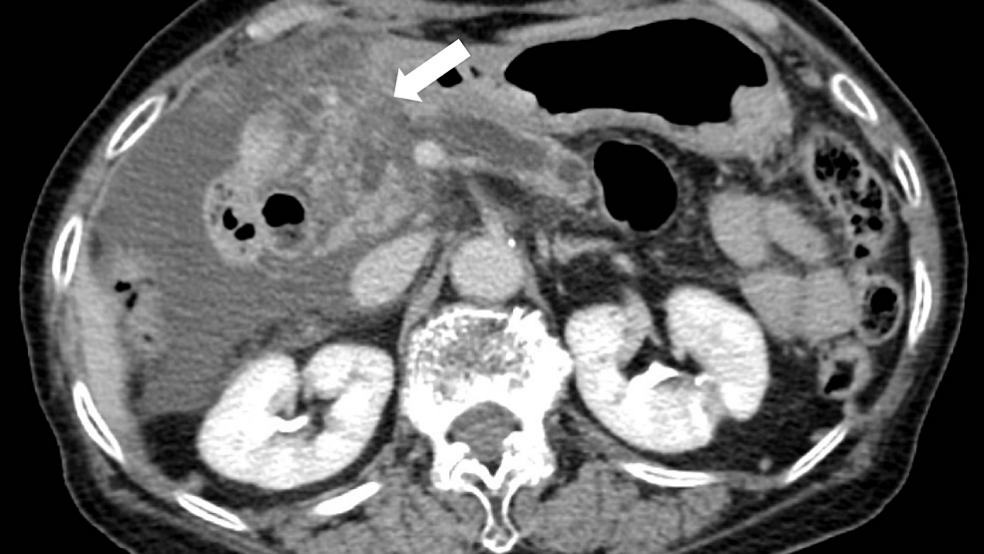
Contrast-enhanced computed tomography image of the abdominopelvic region showing an enlarged pancreatic head (white arrow)
